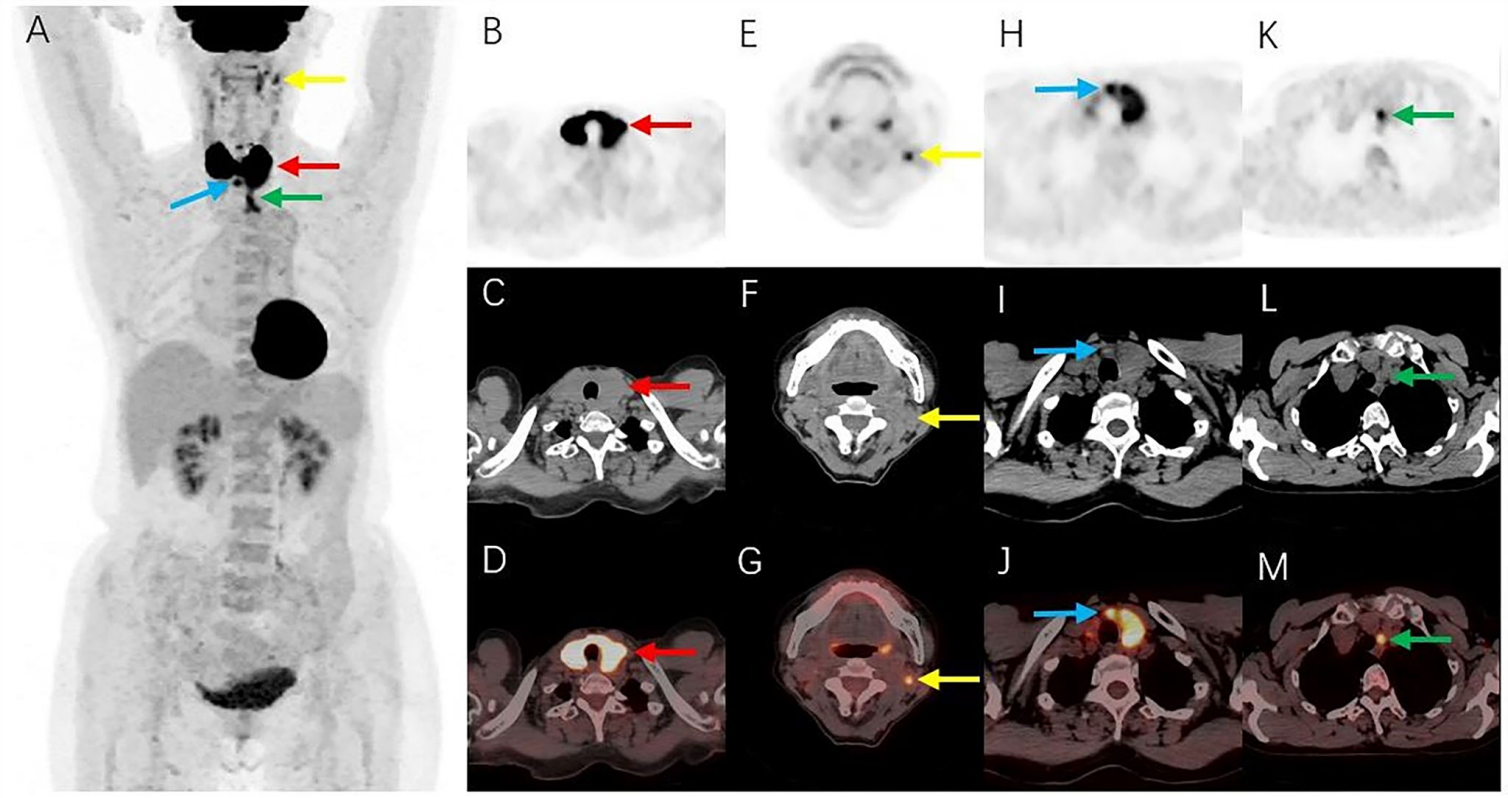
[^18^F]FDG PET/CT images. (A) Maximum intensity projections showed intense FDG uptake in both thyroid gland lobes (red arrow). Localized FDG uptake was also observed in the left level IIa (yellow arrow), level VI (blue arrow), and level VII (green arrow) of the neck. (B–D) Axial images (B, PET images; C, CT images; D, fusion images) showed intense FDG uptake in both thyroid gland lobes (red arrows), with diffuse enlargement and reduced density of both lobes on a corresponding CT scan. (E–M) Transverse PET (E, H, K), CT (F, I, L), and fusion images (G, J, M) showed lymph nodes with moderate FDG uptake in the left level IIa (yellow arrows), level VI (blue arrows), and level VII (green arrows) of the neck. [^18^F]FDG, 2-Deoxy-2-[^18^F]fluoro-D-glucose; PET/CT, positron emission tomography/computed tomography.
